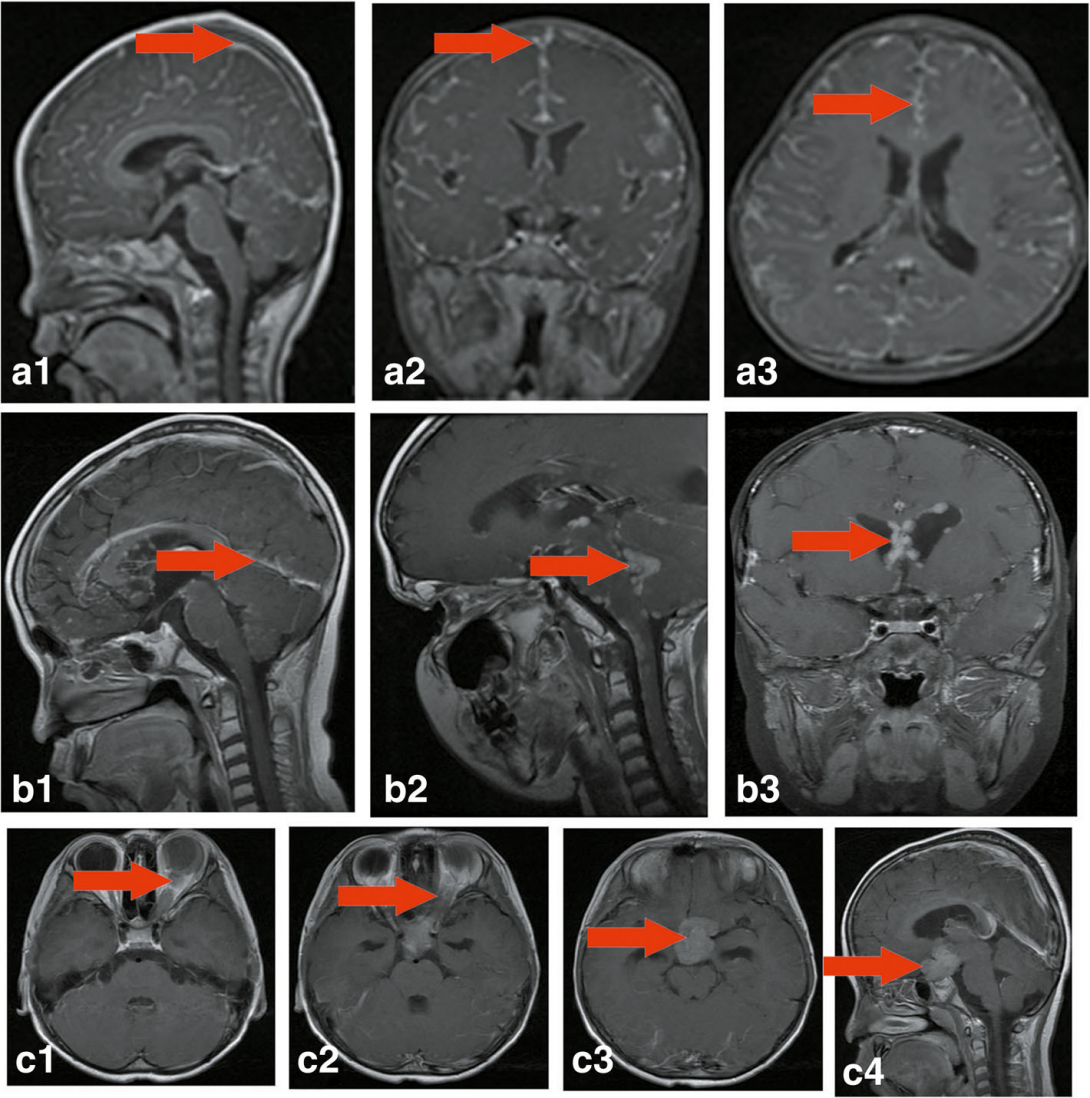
Representative MRI results of retinoblastoma patients with CNS metastasis. (a1–3) Head contrast-enhanced MRI: visible diffuse enhancement of the intracranial pial from sagittal, axial, and coronal imaging, respectively, cervical spinal cord membrane also show enhancement. (b1–3) Head and orbital contrast-enhanced MRI: sagittal image shows thickened meninges of the anterior, middle and posterior cranial fossa, brain stem surface, and the cerebellum (b1); and orbital MRI images show enhanced signaling of nodules, possibly spreading via the cerebrospinal fluid (b2–3). (c1–4) Head MRI: signs of optical nerve, optic chiasma, and supra sella cistern involvement, respectively (c1–3); visible mass formed in the supra sella cistern, and enhanced signal of the cerebral falx (c4). MRI: magnetic resonance imaging; CNS: central nervous system
